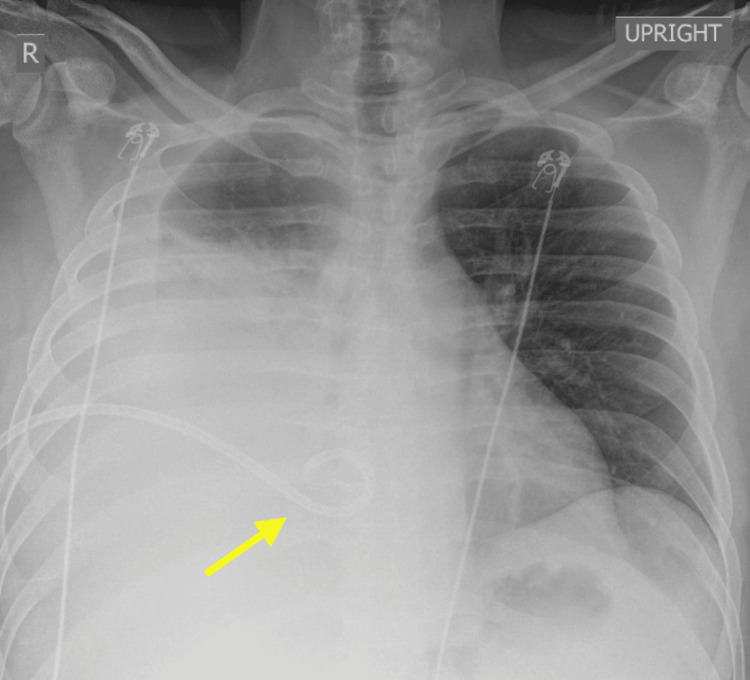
Chest X-ray after pigtail chest tube placement Pigtail chest tube projected over the right lower medial chest (yellow arrow) with a decrease in the pleural effusion noted in the initial chest X-ray.
